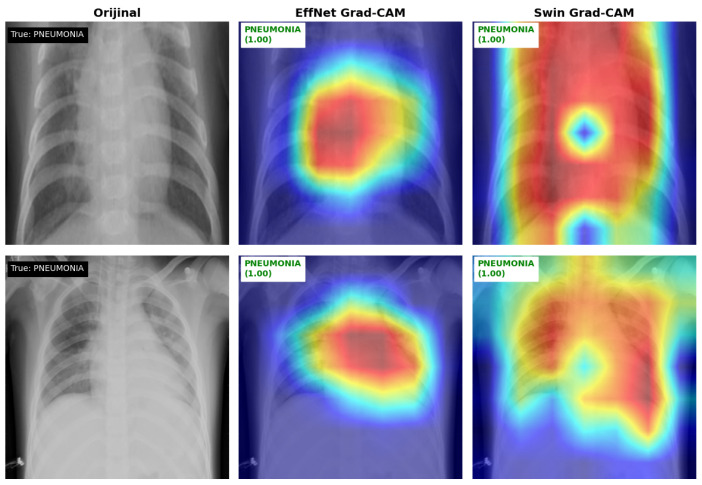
Grad-CAM visualizations of representative pediatric pneumonia chest X-ray images generated by EfficientNetV2-S and Swin Transformer-T. In the Grad-CAM visualizations, warmer colors indicate higher activation, whereas cooler colors indicate lower activation.
